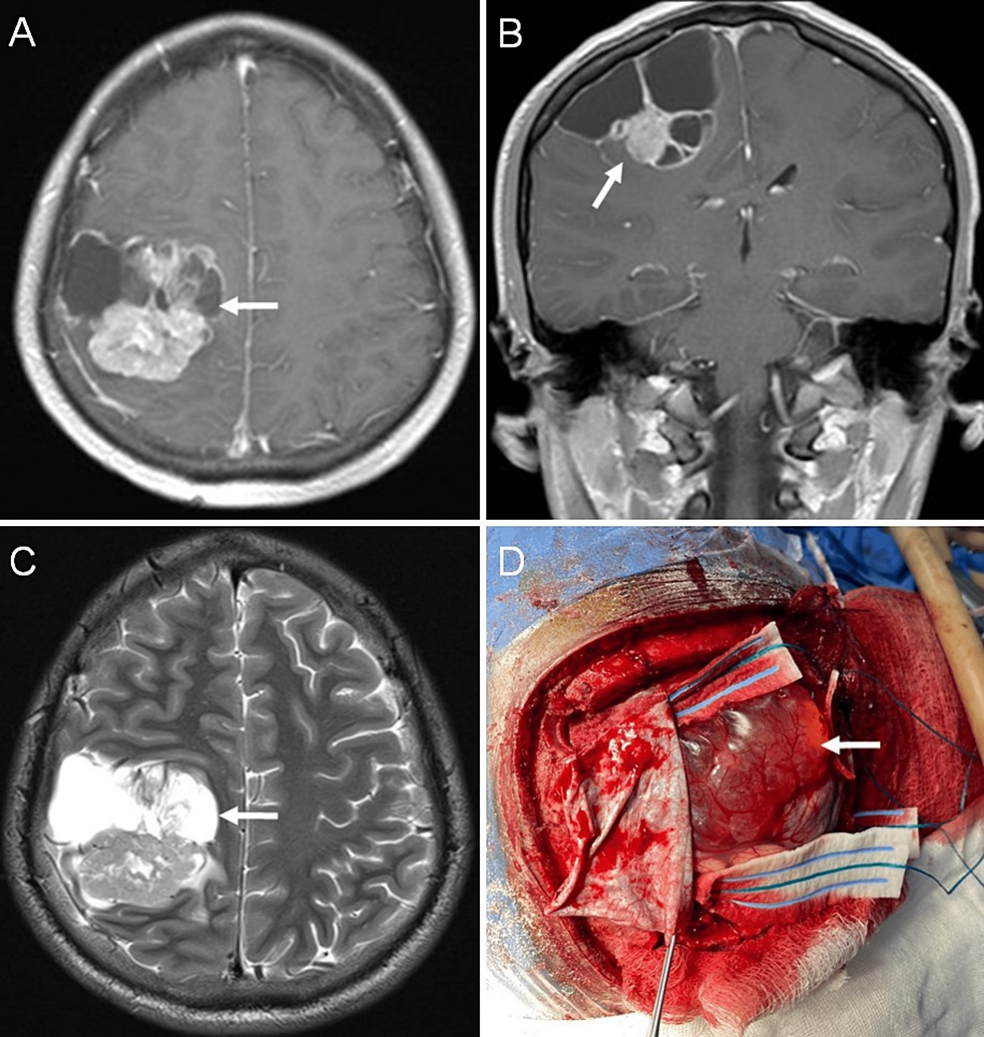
Preoperative MRI images and intraoperative macroscopic surgical view (A, B) Axial T1-weighted MRI section with contrast demonstrating a supratentorial extraventricular lesion (white arrow). (C) Axial T2-weighted section shows solid-cyst component (white arrow). (D) Surgical view shows a cystic cortical lesion after durotomy (white arrow).
